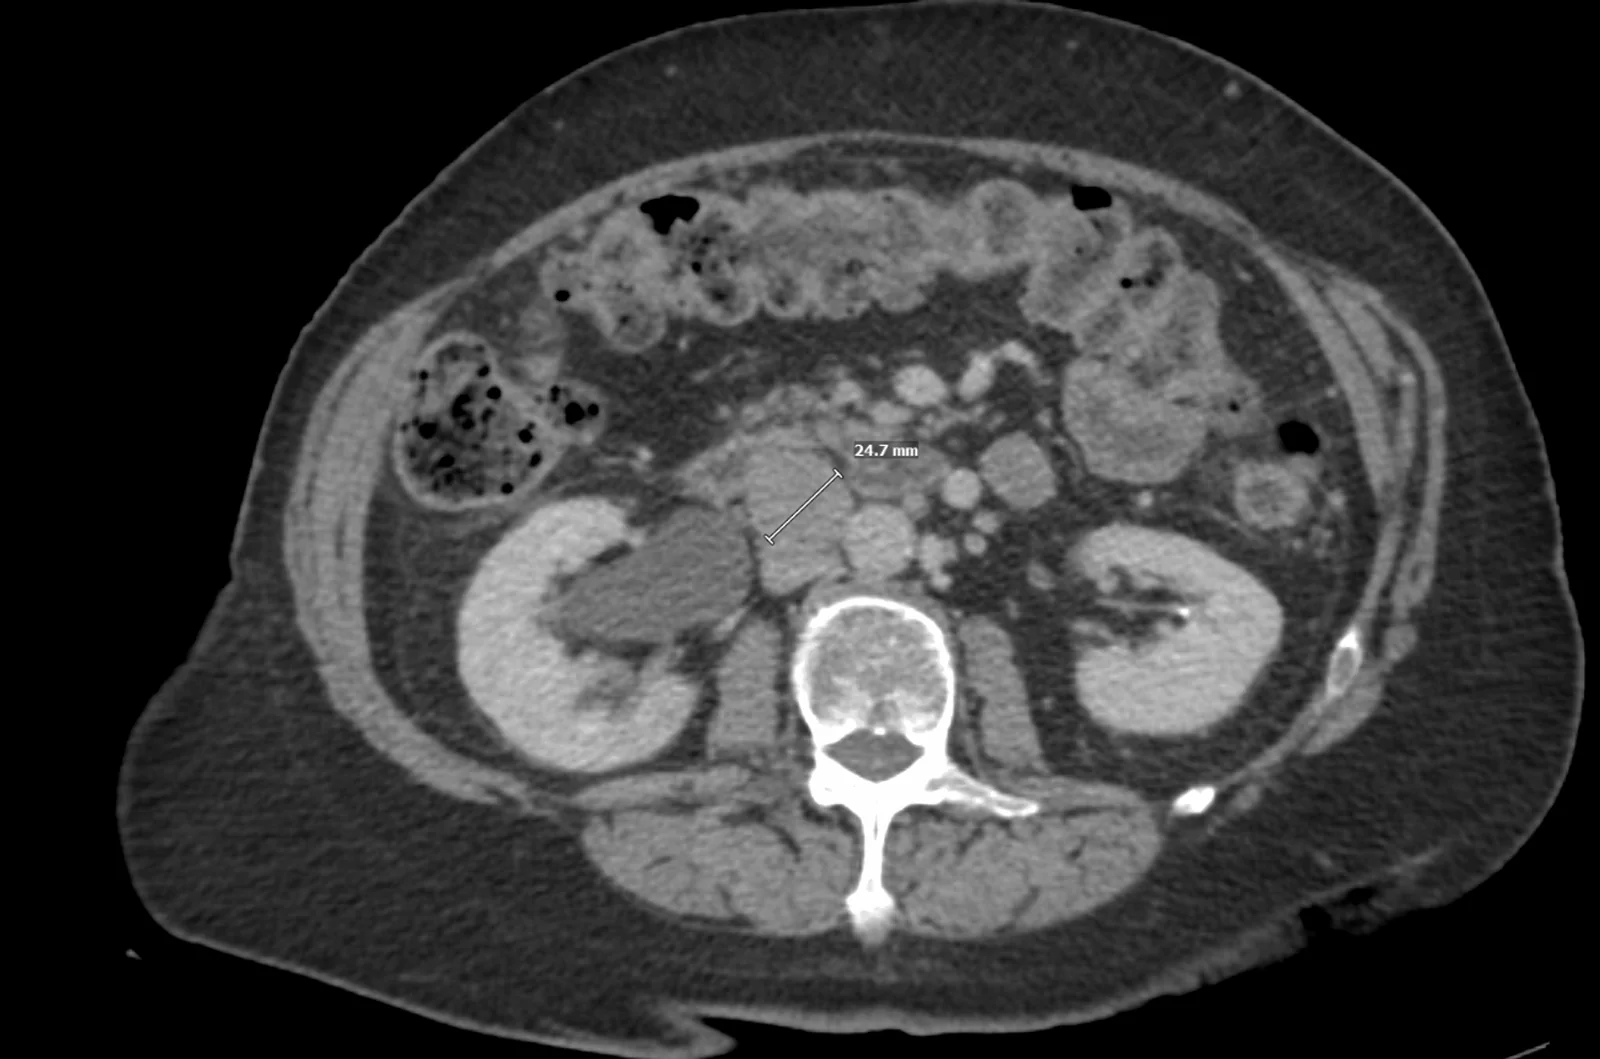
Restaging CT after 6 cycles of panitumumab and irinotecan again showed increased retroperitoneal lymphadenopathy (to 24.7 mm)
